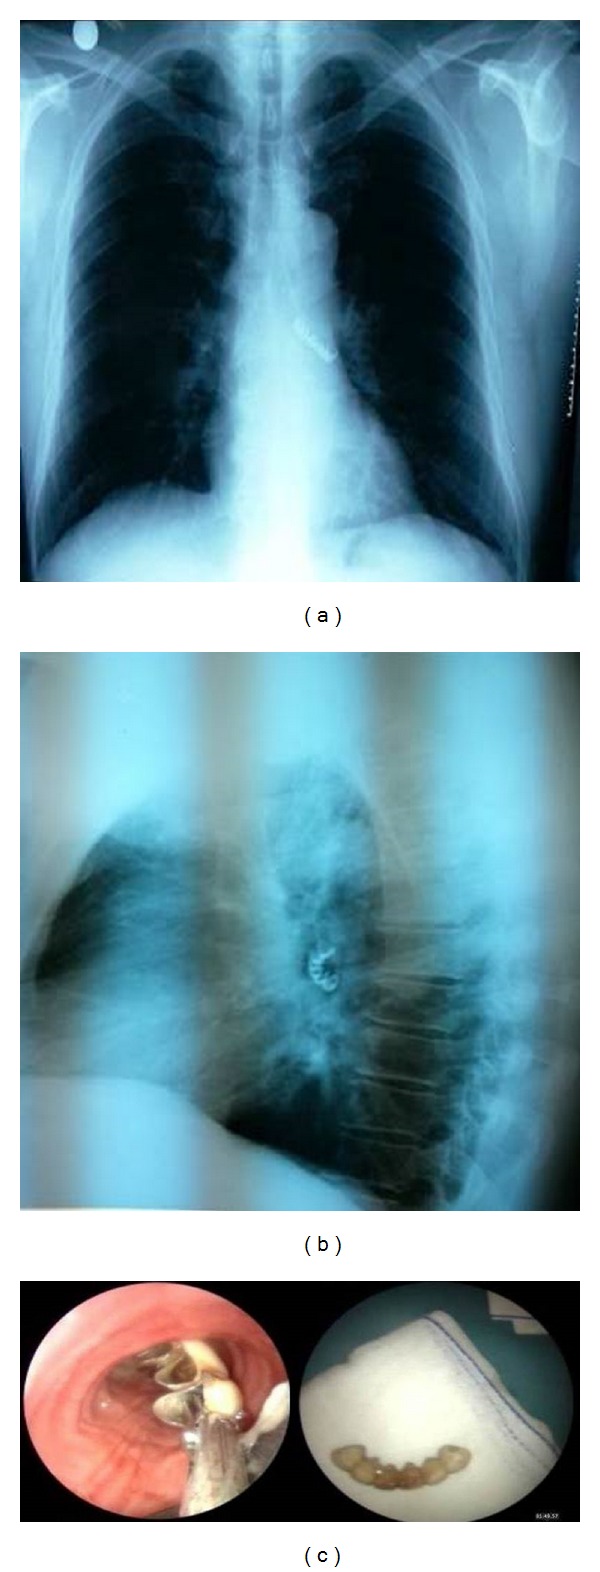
PA (a) and lateral (b) chest X-ray view in the left main bronchus radiopaque foreign body was considered as a dental prosthesis. (c) Extracted dental prosthesis with bronchoscopy.
